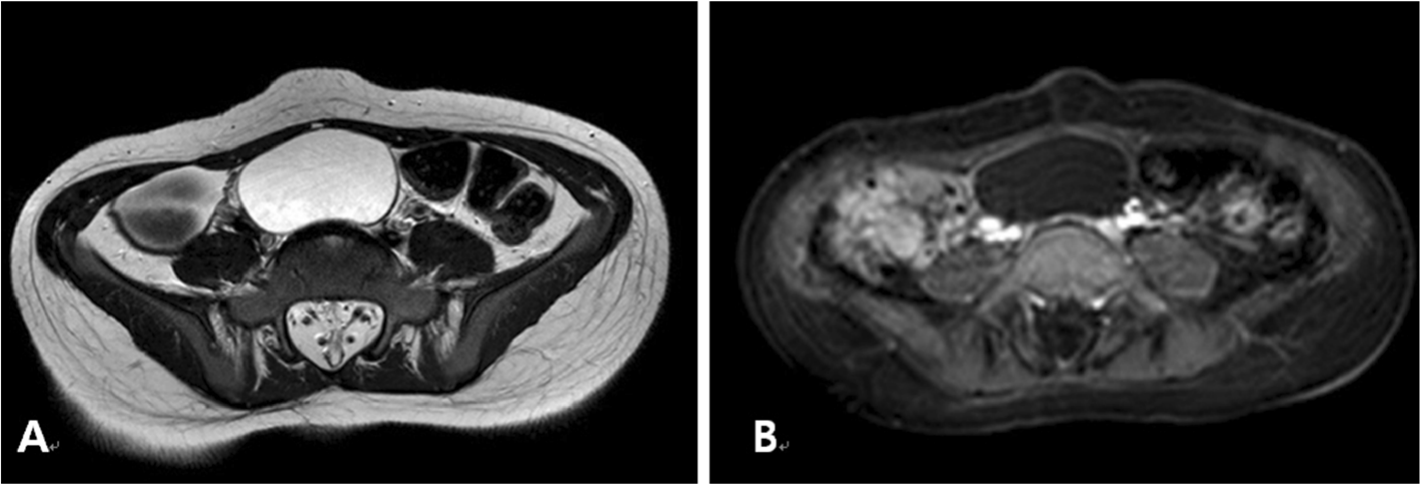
An Axial T2-weighted MR image (A) and T1-weighted contrast enhanced MR image (B) show an ovoid to round cystic mass with well-defined margins and a thin wall without any internal component.
